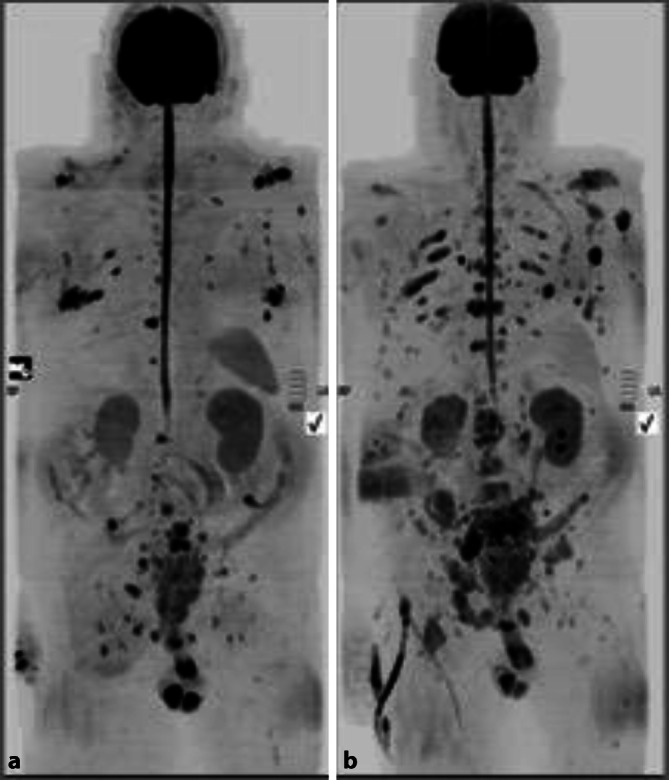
Assessment of disease progression on whole-body magnetic resonance imaging in a 67-year-old male patient with metastatic castrate-resistant prostate cancer on Zoladex and bicalutamide (MAB/CAB). Image a: April 2016, Image b: August 2016 demonstrating multifocal disease progression with increase in the number and the size of the lesions on diffusion-weighted imaging, with nodal, extra-nodal, and metastatic bone disease as well as further locoregional invasion with bladder and pelvic soft tissue invasion. (Courtesy of Prof. Anwar Padhani, © Prof. Padhani. This content is not part of the Open Access License.)
